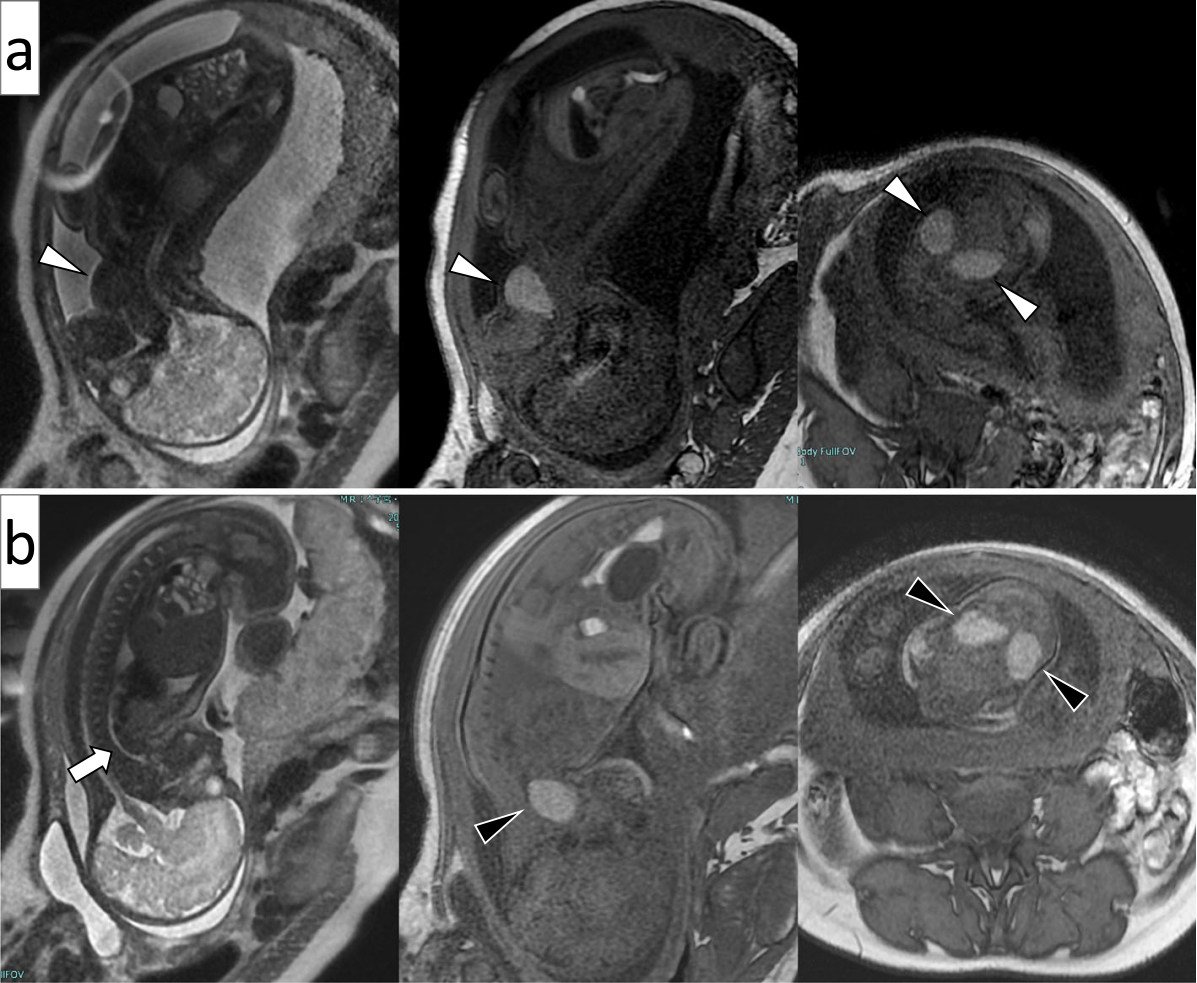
Fetal MRI images. Left: T2-weighted sagittal section of the fetus, Middle: T1-weighted sagittal section of the fetus, Right: T1-weighted transverse section of the fetus. a: Images taken at 34 weeks and 5 days of gestation. The anterior side of the cervical region of the fetus is swollen and protruding, and the fetus is in face presentation. The cervical region of the fetus shows a mass with left-right symmetry. T1-weighted images are hyperechoic and T2-weighted images are hypoechoic (△). b: Images taken at 36 weeks and 3 days of gestation. The cervical mass of the fetus (▲) shows no change in the MRI intensity, although it does show slight tendency toward shrinkage. The fetus is in a normal, flexed presentation, and the volume of amniotic fluid has decreased. A T2-weighted sagittal section of the fetus confirms the fetal airway (⇧)
